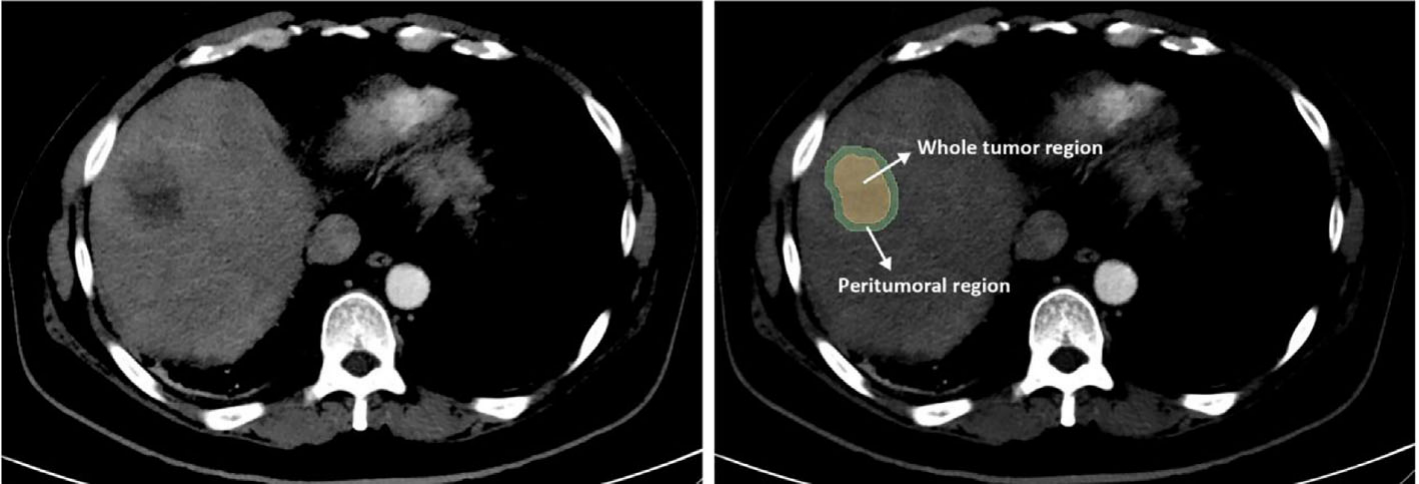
Image segmentation of radiomics. The whole tumor region (WTR) was manually segmented along the contour of the tumor on the axial slice of plain and contrast-enhanced CT. Then, a region with 10 mm distance to the tumor contour was automatically created by the 3D Slicer software and defined as the peritumoral region (PTR).
